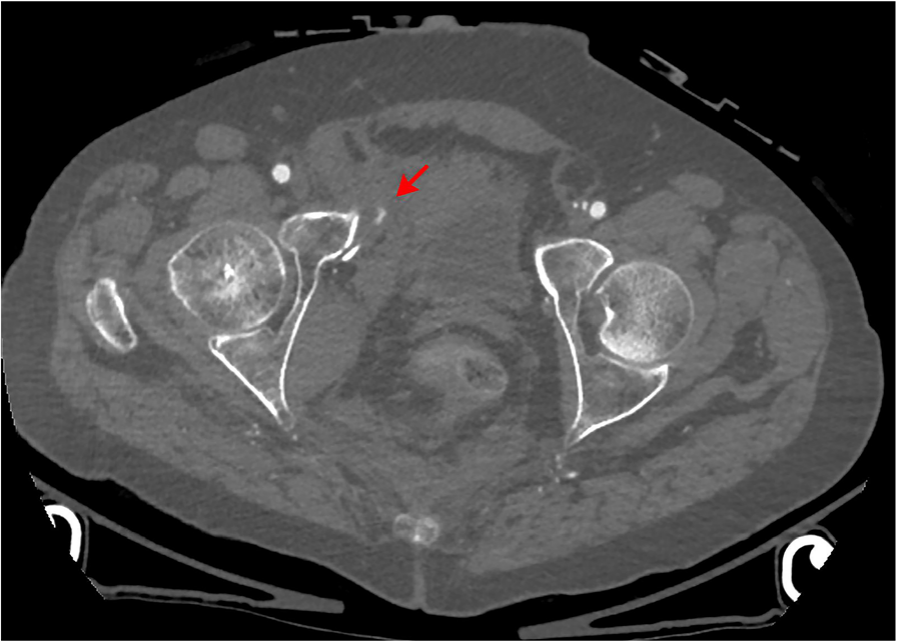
A 64-year-old polytrauma female patient admitted with reduced consciousness, pelvic fracture dislocation and right pelvic haematoma. Urgent arterial phase trauma CT scan (1 mm slice thickness) axial image showing a high-density focus (red arrow) representing contrast extravasation in keeping with active bleeding, see online Additional file 1
